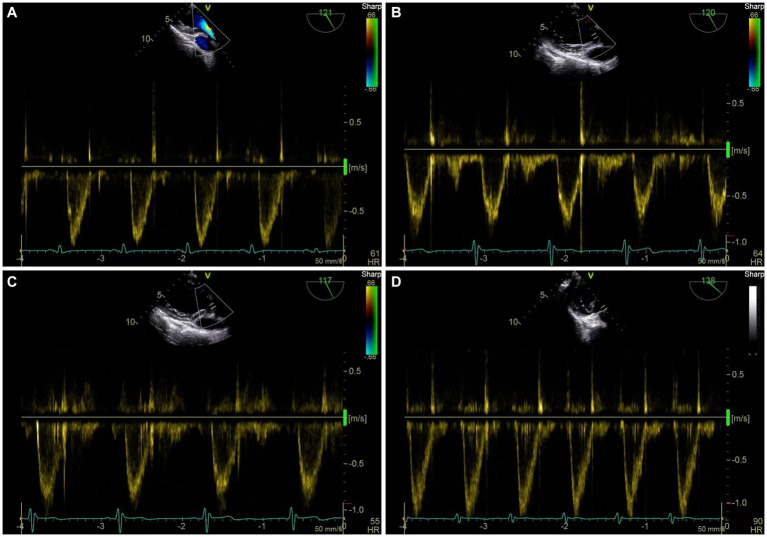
Representative figure of peak systolic velocity at aortic valve before and after VTC and volume expansion as measured by transesophageal Doppler ultrasound. (A) Systolic blood flow spectrum of left ventricular outflow tract (LVOT) at aortic valve orifice at T1. (B) Systolic blood flow spectrum of LVOT at aortic valve orifice at T2. (C) Systolic blood flow spectrum of LVOT at aortic valve orifice at T3. (D) systolic blood flow spectrum of LVOT at aortic valve orifice at T4.
